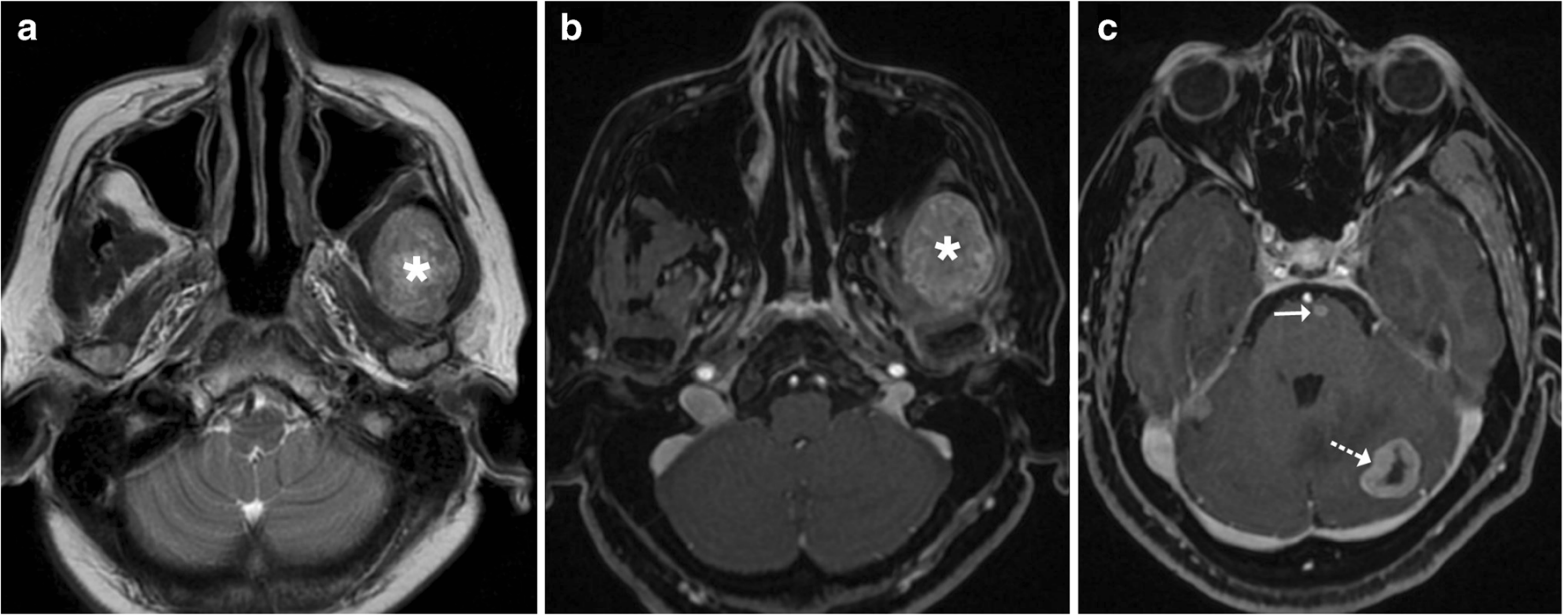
A 17-year-old patient with acute myeloid leukaemia: a–b Axial T2 and post-contrast T1-weighted image shows an enhancing mass in the left masticator space suggestive of a chloroma (*asterisk*). c Axial T1-weighted image shows enhancing deposits in the left cerebellum (*dotted arrow*) and pons (*arrow*)
